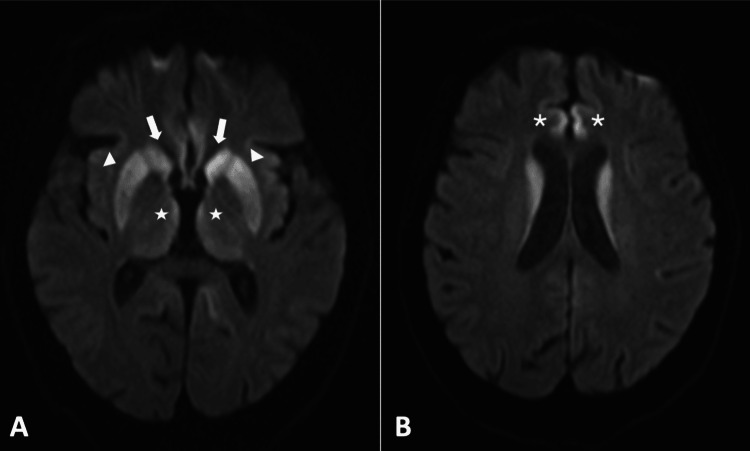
Neurodegenerative process associated with CJD: cranial MRI findings (Case 1). Subsequent axial cranial MRI diffusion-weighted imaging (DWI) sequence, showing restricted diffusion in both caudate nuclei (wide arrow), the putamen (arrowhead), internal and posterior surfaces of the thalami (star), and bilateral superior internal frontal gyrus (asterisk). CJD, Creutzfeldt-Jakob disease; MRI, magnetic resonance imaging
